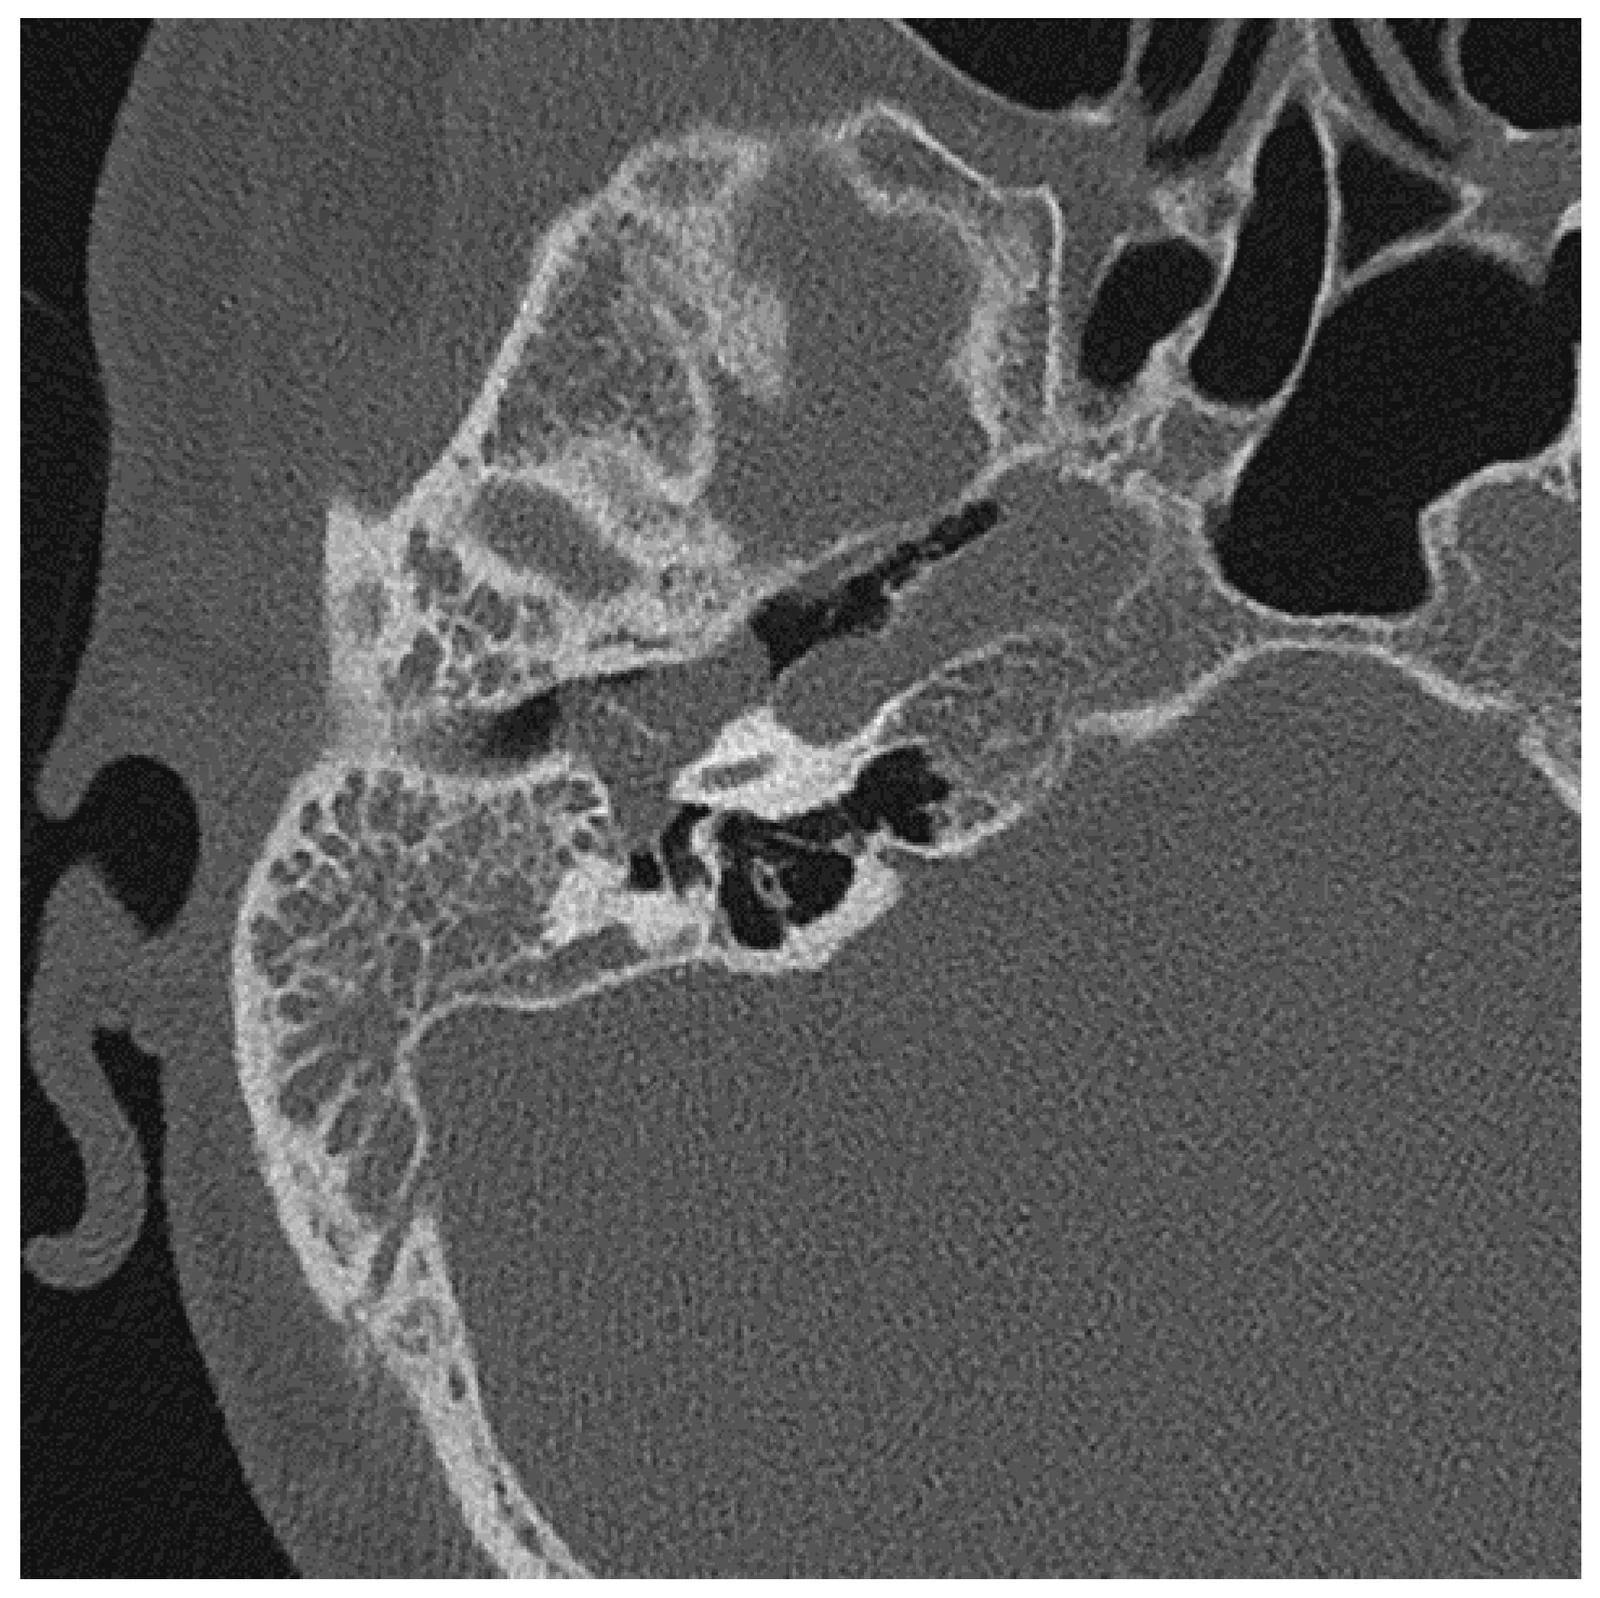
CT scan of the right ear.
